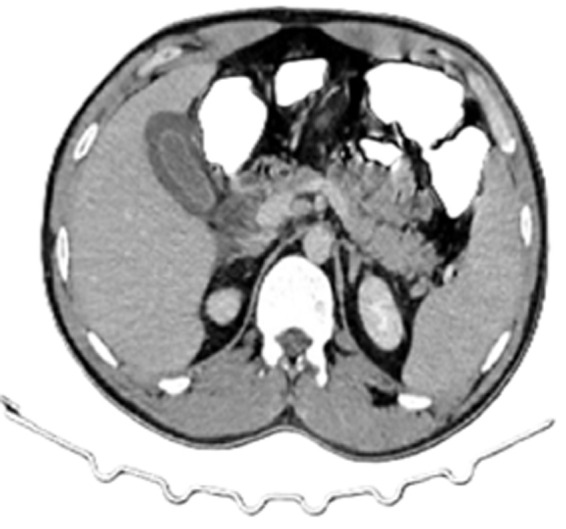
axial computed tomography image demonstrating a thickened gallbladder wall suggestive of ischemic changes
